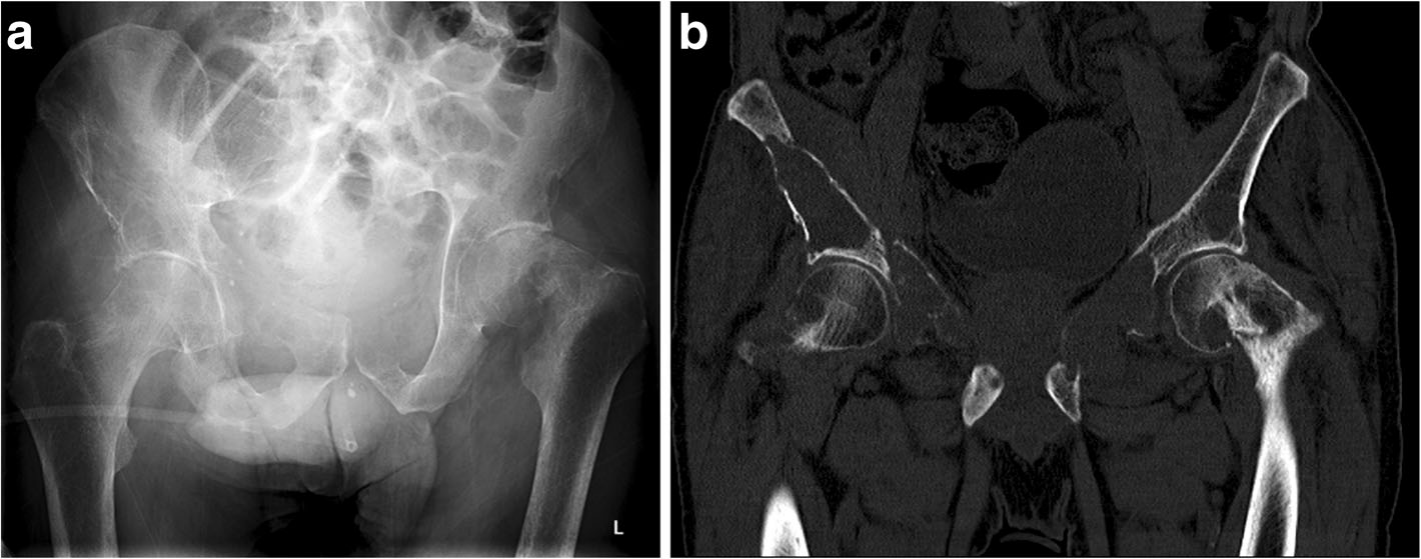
a, b Patient presented after a fall. The pelvic radiograph showed a left subcapital neck of femur fracture with a lytic area in the inferior aspect of the femoral head. Coronal CT showed multiple lytic areas
